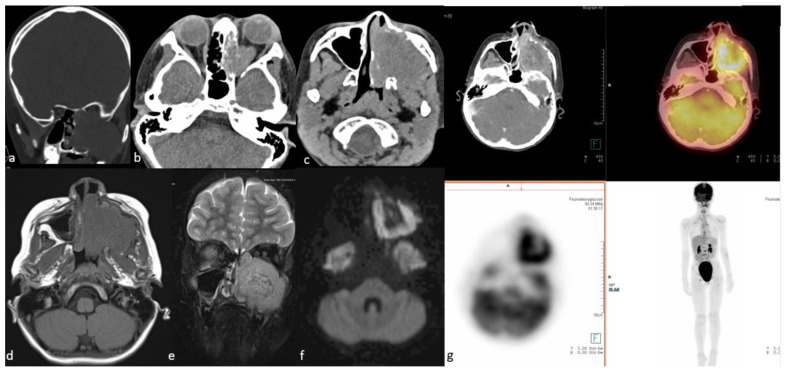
Pediatric sinonasal alveolar rhabdomyosarcoma. A 9-year-old female patient presenting with persistent left nasal drainage. Initial CT (a–c) shows an infiltrative soft tissue mass originating from the left maxillary sinus and nasal cavity with erosive changes in maxillary sinus walls and extension into the left masticator space and left extra/intraconal spaces. Subsequent MRI confirms the same findings (d–f) and shows increased signal on DWI (f), indicating high cellularity. The central portion of the lesion is necrotic. ^18^F-FDG PET/CT (g) again shows the central necrotic changes and, in addition, shows uptake in multiple lytic axial skeleton lesions. A biopsy revealed an alveolar rhabdomyosarcoma.
